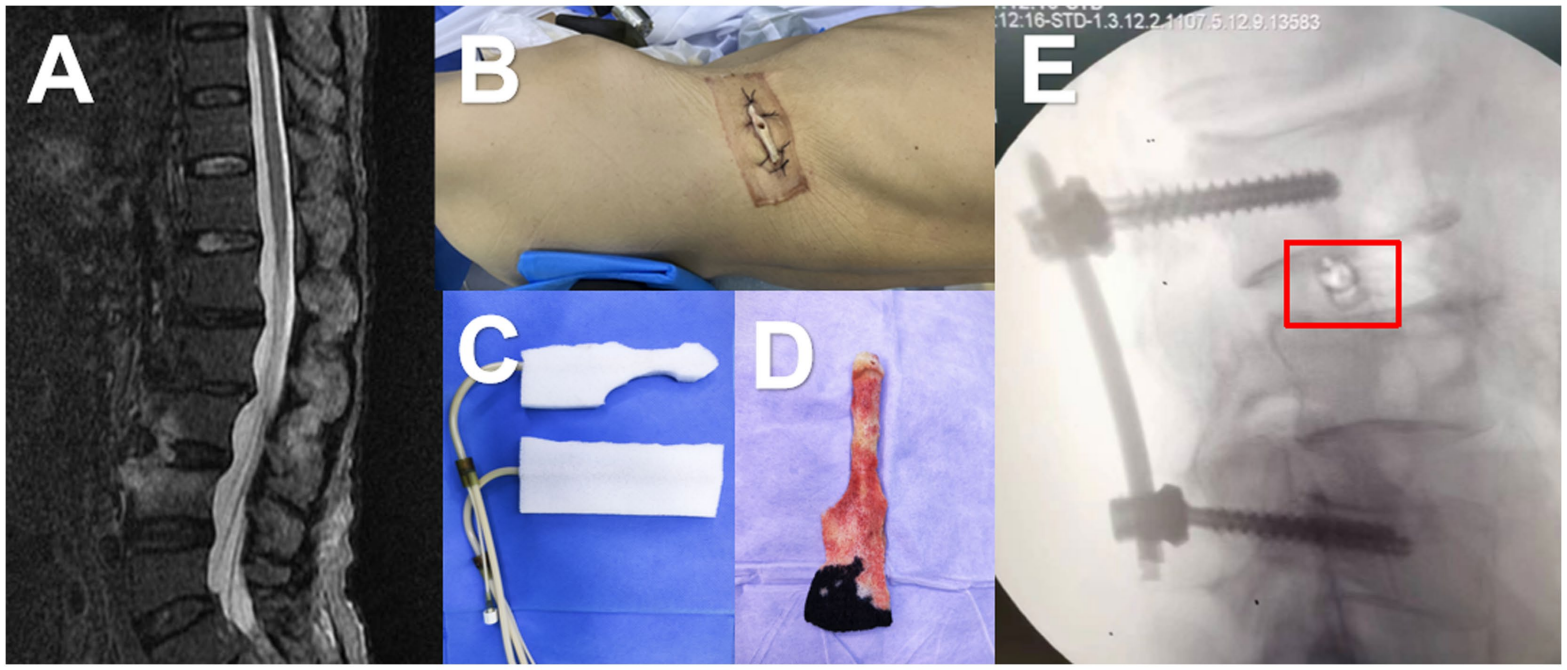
A 68-year-old male was admitted to the hospital with a month-long history of low back pain and fever. (A) MRI revealed increased signals on the anterior and inferior margins of the L3 and L4 vertebrae, as well as T2 liposuppressive images in the L3/4 space. (B) The patient was then placed in a lateral position and underwent a small incision debridement. (C,D) A negative pressure drainage sponge was inserted into the intervertebral space, and the negative pressure was connected. (E) Intraoperative fluoroscopy showed that the sponge was in the correct position (marked in red box).
